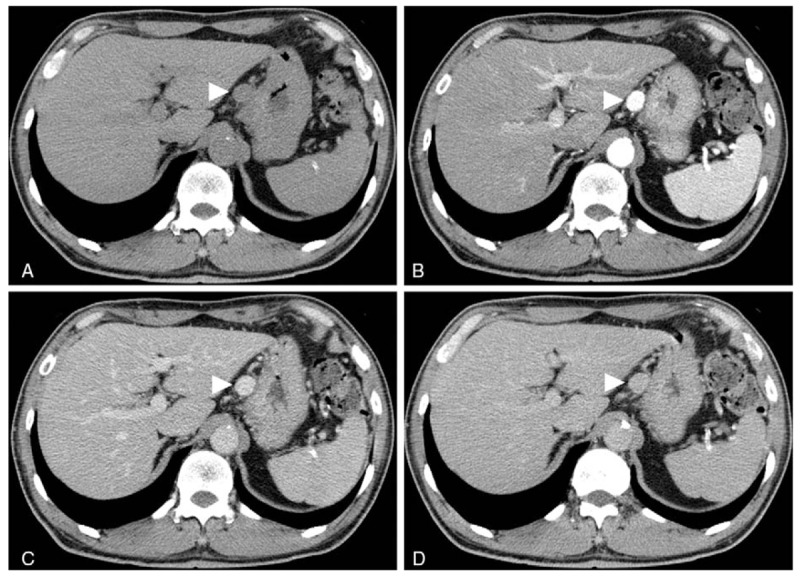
Contrast-enhanced CT revealed a round-shaped tissue mass that was 18 × 15 mm by the lesser curvature of the stomach. A: Plain CT showed a tissue mass (arrowhead) without calcification. B: The early phase of enhanced CT revealed a well-circumscribed mass (arrowhead), which showed a homogenous, highly enhanced effect without communication with the gastric wall. C: The image obtained at 120 s after the injection of contrast medium showed attenuation of the mass (arrowhead), but the contrast effect was still present. D: The image obtained at 5 min after the injection of contrast medium showed isodensity of the mass (arrowhead) compared with the liver and spleen. CT = computed tomography.
